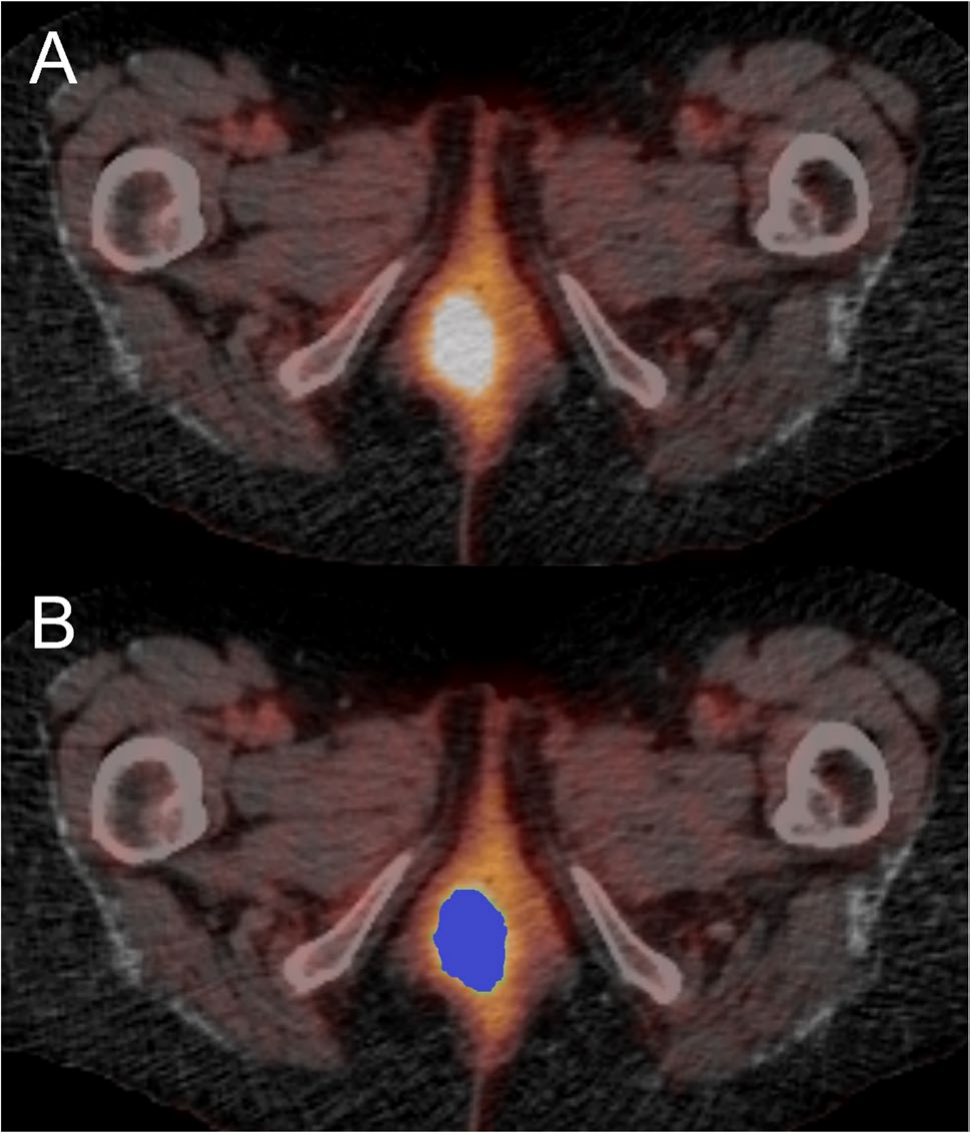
Axial PET-CT slices demonstrating a primary anal carcinoma mass (A) and the overlying mask created from a 1.5 times mean liver SUV (B)
